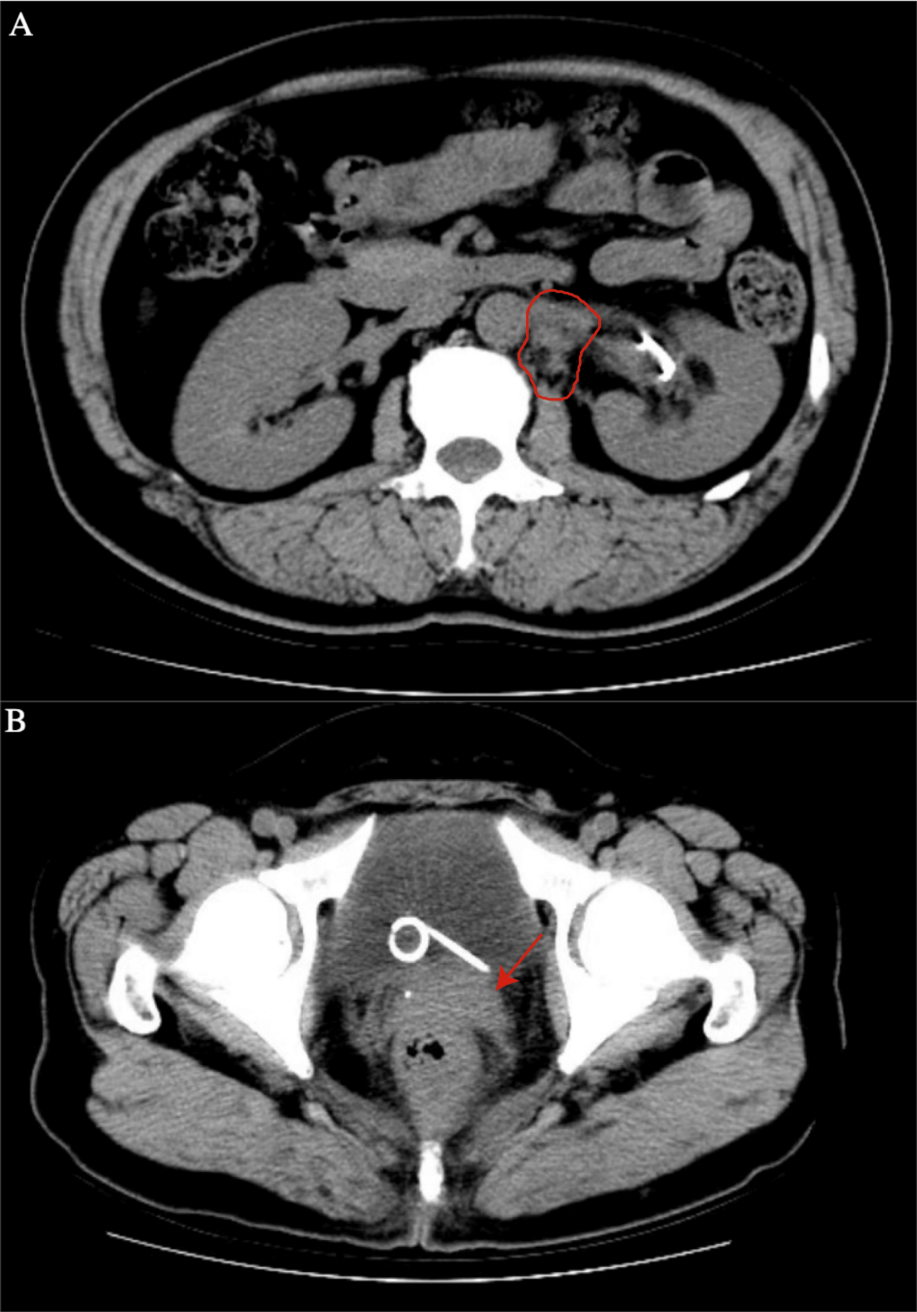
Enhanced computed tomography scan of the abdomen and pelvis following two cycles of idarubicin and cytarabine-based chemotherapy, showing a significant reduction in the previous mass. (A) Blurred fat planes are seen surrounding the left renal hilum and upper ureter, with a mass (outlined by the red line) visible at the level of the left renal hilum in the retroperitoneum. The mass shows poorly defined borders with the abdominal aorta, left renal artery, and left psoas muscle. (B) The cervix appears enlarged, with slightly obscured fat planes in the surrounding tissue (indicated by the red arrow).
